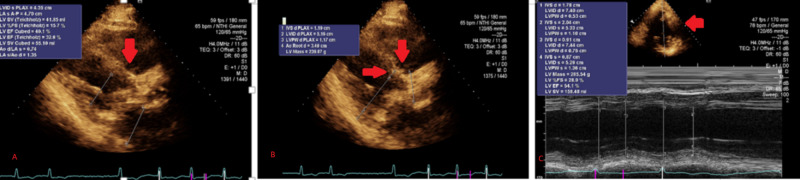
Echocardiogram showing severe aortic stenosis (A, B) Parasternal long-axis view with red arrows indicating a severely stenosed aortic valve with minimal opening in systole. (C) Echocardiogram with M-mode showing minimal opening of the aortic valve in systole and narrow valvular orifice consistent with severe aortic stenosis.
